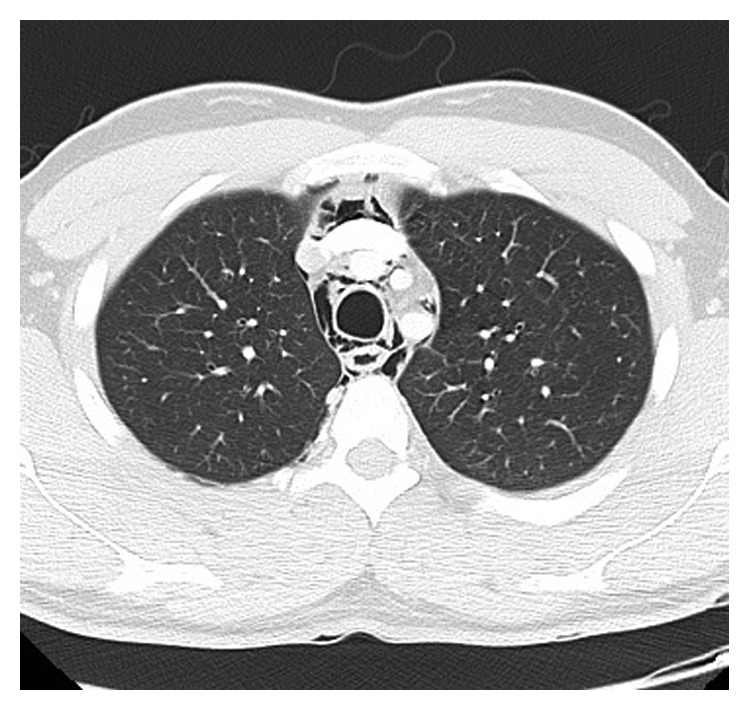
Axial CT showing free air surrounding mediastinal structures.
